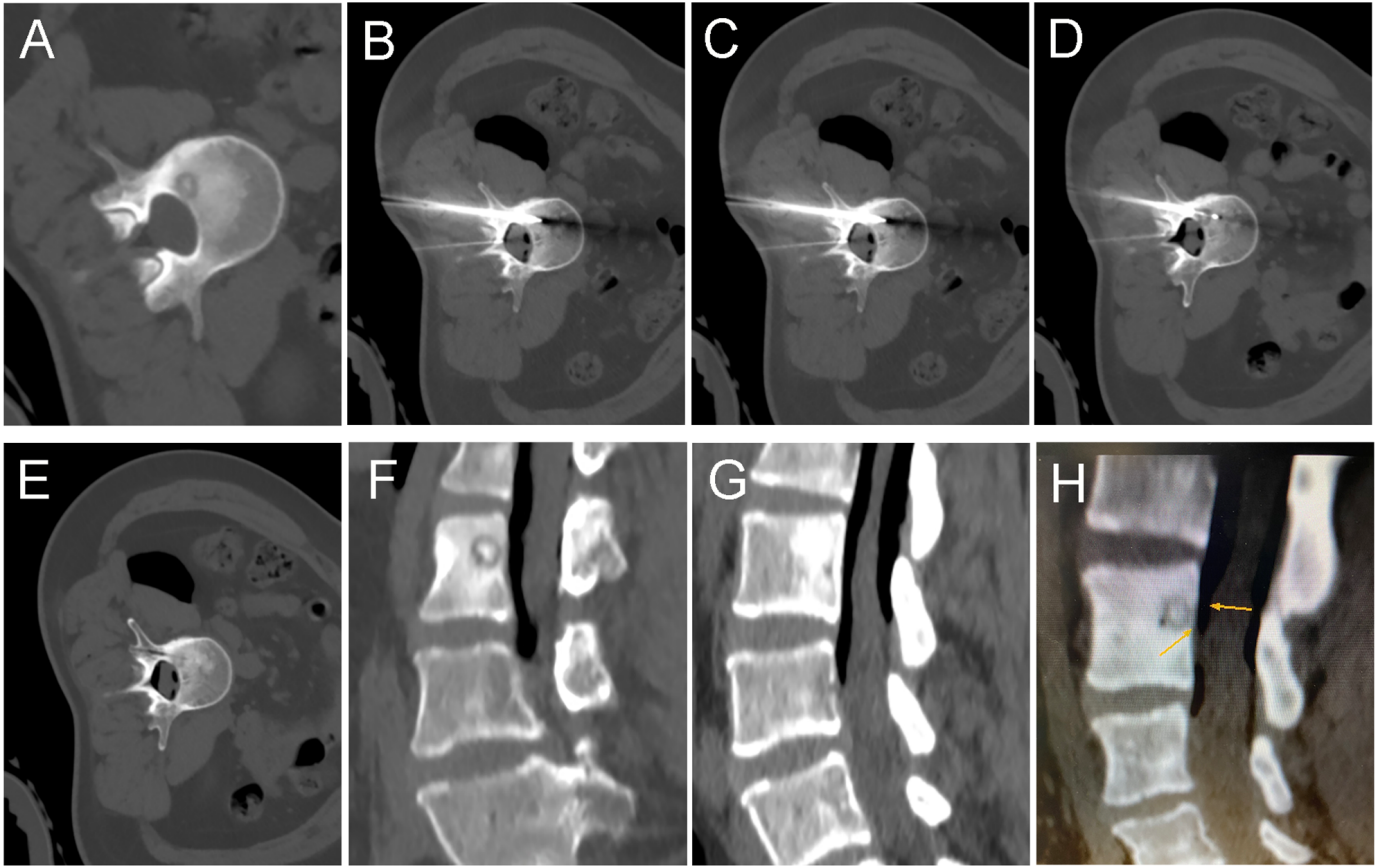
A 35-year-old female with intermittent low back pain for more than six months. (A) Pre-operative CT showed a round lower density lesion on the lumbar three vertebrae, with a dotted high-density nest in the center. (B, C, D) A cryoprobe was passed from the back through the appendages to reach the vertebral foci. At the same time, a 19G fine needle was used to puncture the epidural space, and a syringe was used to inject filed air into the spinal canal epidural space. (E) After the freezing was completed and the frozen needle was removed, a repeated CT scan should be performed to exclude complications. The needle path was visible. (F, G, H) Sagittal reconstruction images showed the vertebral body successfully isolated by air between the lesion and the dural sac. The air outside the dural sac protected the structures in the spinal canal from damage while freezing at full power.
